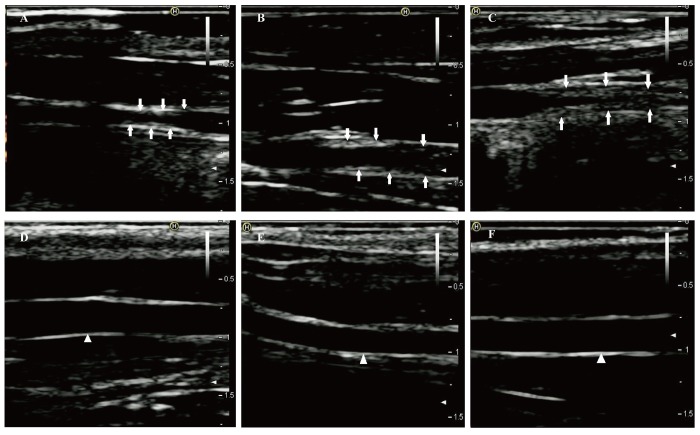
Two-dimensional ultrasound of right common carotid arteries at eighth week. (A-C) Two-dimensional ultrasound images show that the right common carotid arteries in the case group formed obvious atherosclerotic plaques indicated by the arrows. (D-F) Two-dimensional ultrasound images clearly show that the intimas of the right common carotid arteries in the control group remain smooth (white triangle).
